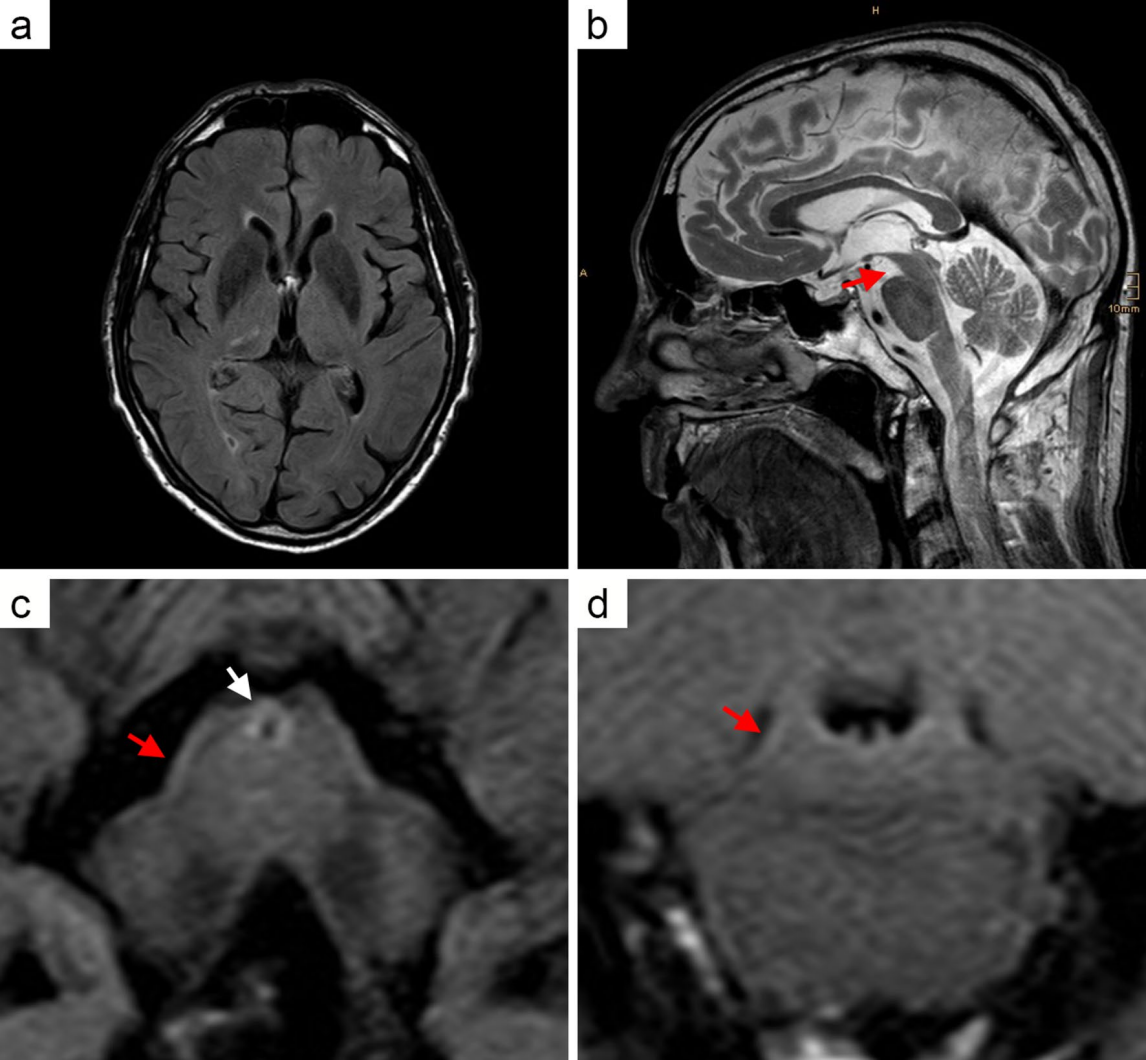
Brain MRI scan of patient 1 with PSP neuropathology. Brain MRI scan (axial, fluid-attenuated recovery sequences (FLAIR)) performed 8 years after symptom onset shows cortical and subcortical atrophy (a). Sagittal FLAIR image reveals reduced size of the mesencephalic tegmentum leading to the hummingbird appearance of brainstem (b; red arrow). Axial FLAIR image of the brainstem shows an increased signal hyperintensity of the periaqueductal grey matter (c, white arrow). Furthermore, atrophy of the mesencephalic and pontine tegmentum is evident (c, d; red arrows)
